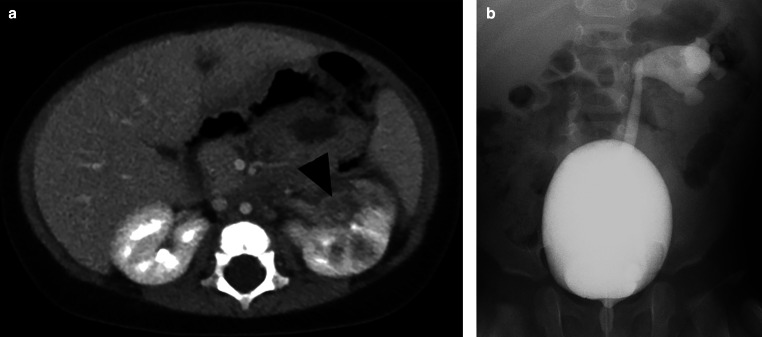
A 6 month-old boy who presented with fever, intermittent fussiness, and leukocytosis (39,000 WBC/mL) was initially evaluated with US for intussusception. Urinalysis was negative on admission and later urine culture was positive for *E. coli.* CT of the abdomen was obtained to evaluate for a mass or inflammatory process; a diagnosis of pyelonephritis was not considered. Axial post-contrast CT (A) shows diffuse swelling and multifocal hypodensities in the left mid-pole kidney, and a round hypodensity in the anterior cortex with wall enhancement compatible with an abscess (arrowhead). A voiding cystourethrogram (B) demonstrates left grade 5 vesicoureteral reflux with a droopy lily sign compatible with a reflux to a lower pole of a duplex kidney. Due to recurrence of UTIs, he subsequently had circumcision and was placed on daily antibiotics. A left ureteral reimplantation was performed after he developed recurrent pyelonephritis
